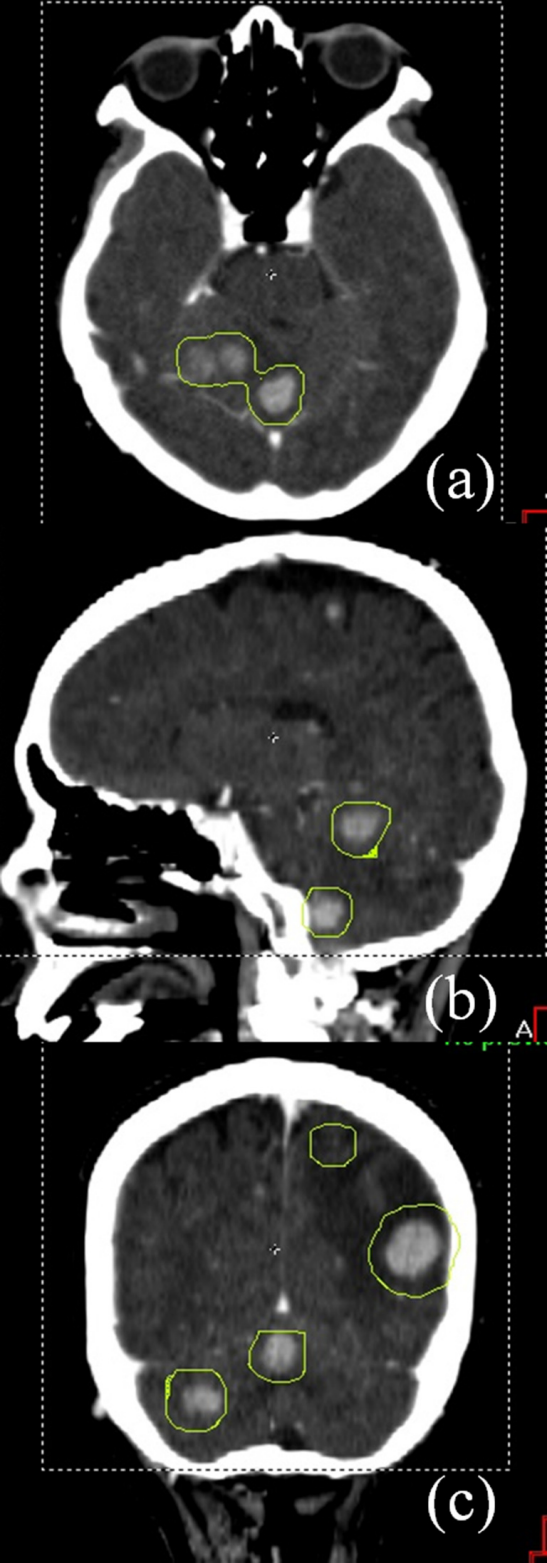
Contrast-enhanced planning CT images on (a) axial, (b) sagittal and (c) coronal planes, with eight lesions contoured as high-risk gross tumour volumes. Planning target volume margins were 2 mm for the targets located within 5 cm from the isocentre, and 3 mm for the other targets located within 10 cm from the isocentre. The resulting planning target volume contours are shown in light green colour.
